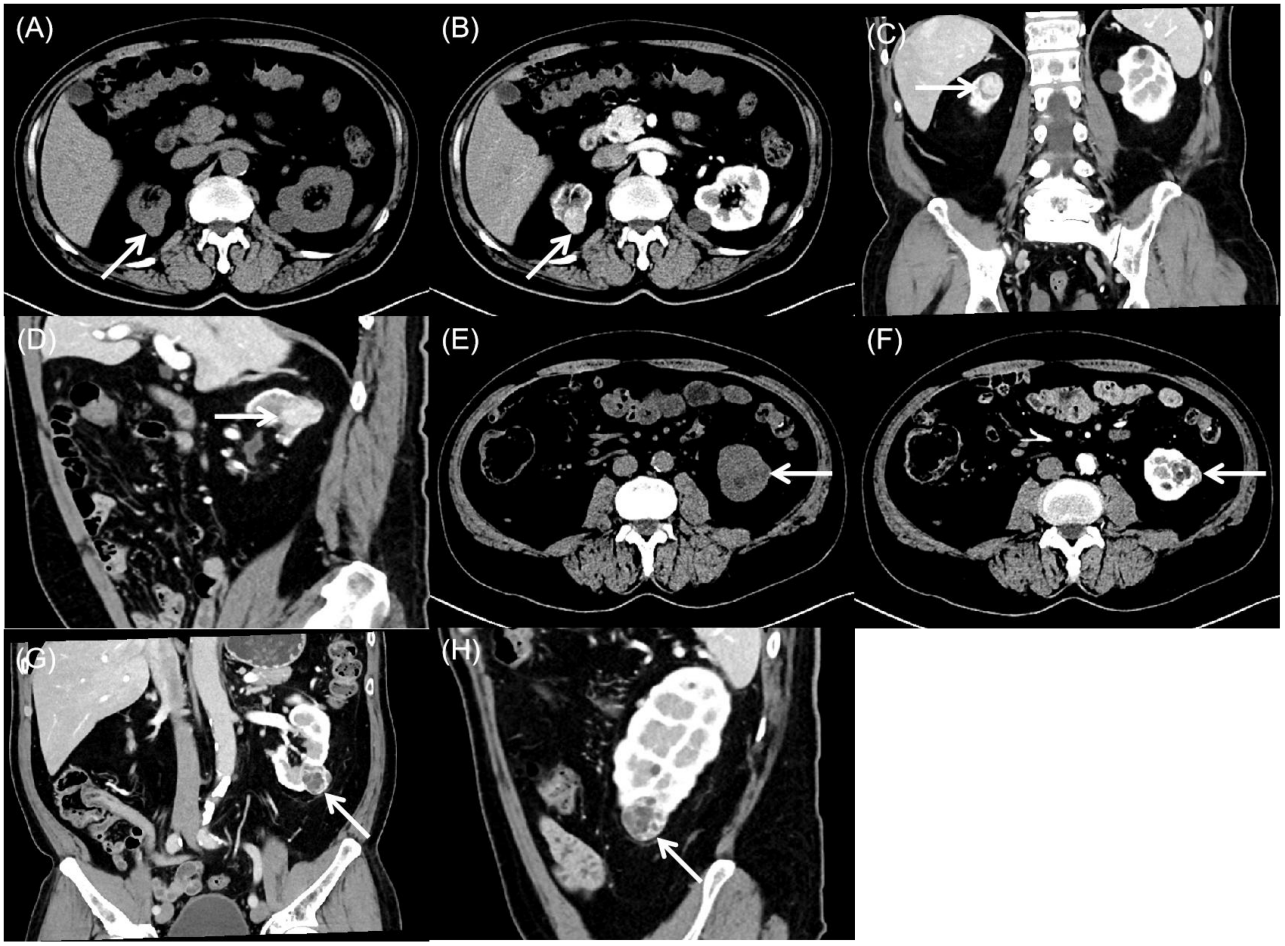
Computed tomography of bilateral synchronous rare renal tumors. (A) Plain computed tomography showed an exophytic, round, heterogeneous soft-tissue attenuation mass (arrow) in the right kidney. (B-D) Contrast-enhanced computed tomography showed marked heterogeneous enhancement during the cortical and medullary phases, with round hypoenhancing foci. Enhancement decreases in the excretory phase while the lesion maintains well-defined margins and persistent internal heterogeneity (arrow). (E) Plain computed tomography showed a well-circumscribed, heterogeneous mass (arrow) with mixed attenuation in the left kidney. (F-H) Contrast-enhanced computed tomography showed characteristic peripheral and septal enhancement, with progressive contrast retention in the delayed phase (arrow).
